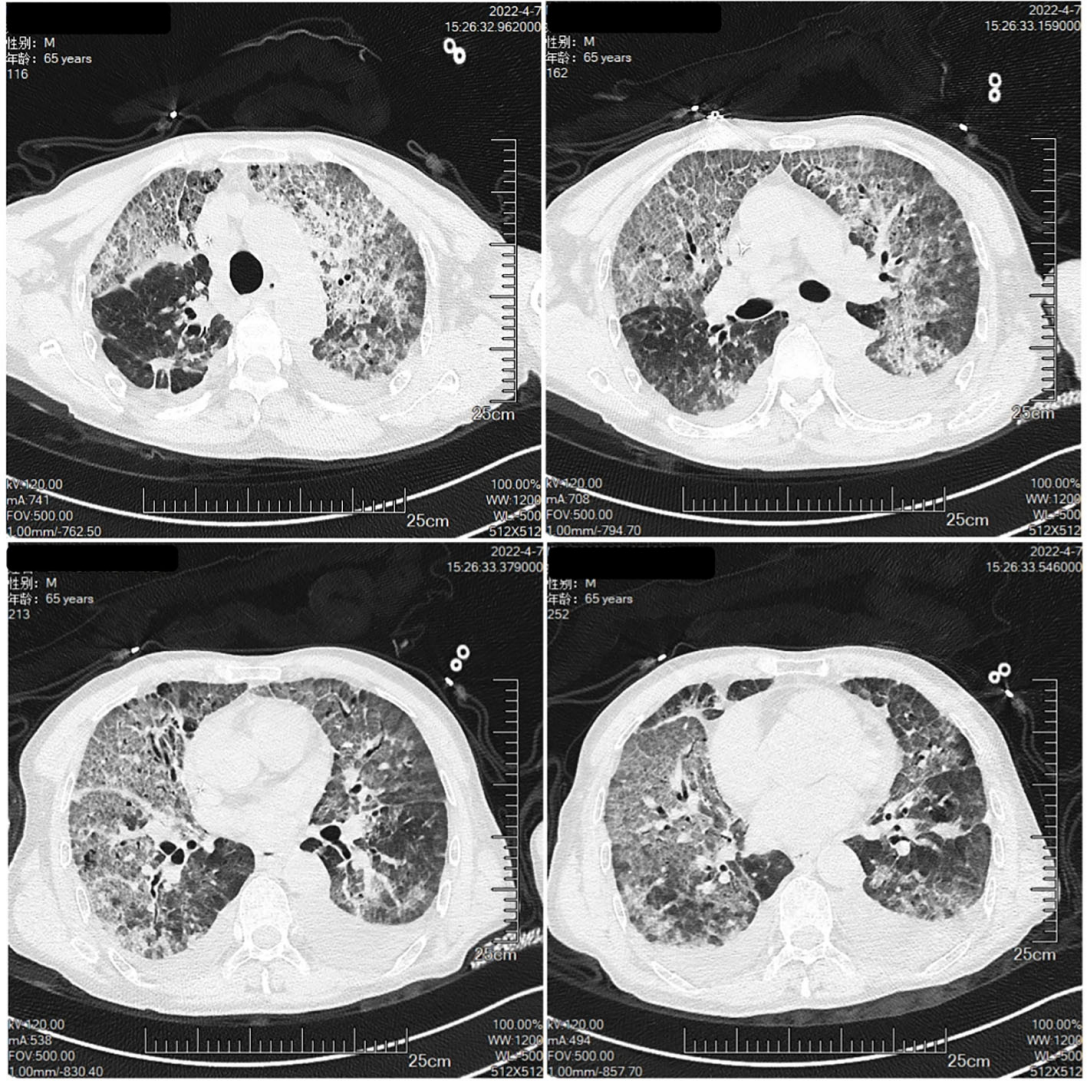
The Chest CT on April 7th.
